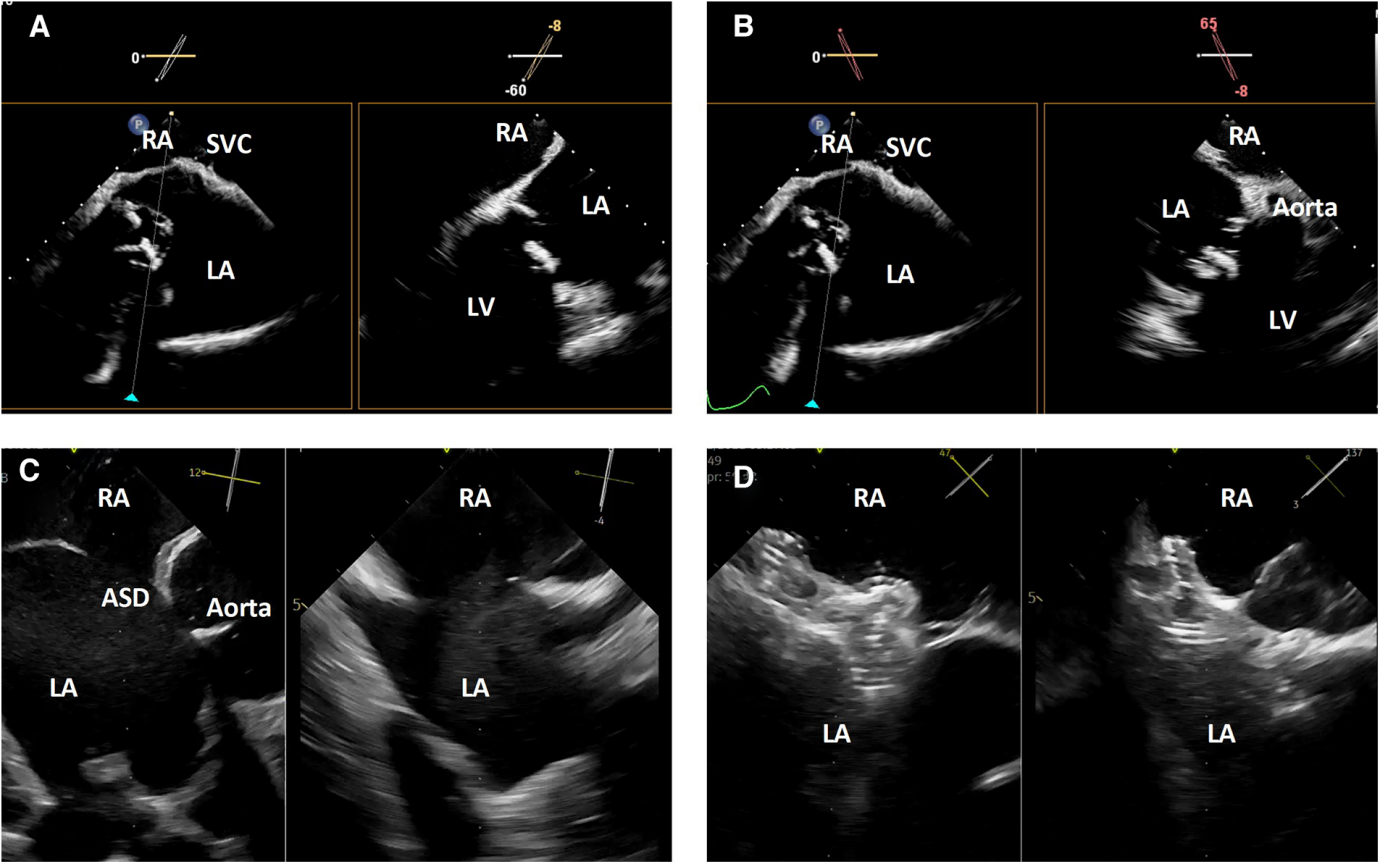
3-dimensional intracardiac echocardiography for transcatheter atrial septal evaluation and interventions. (A) Biplane intracardiac echocardiography evaluation of the interatrial septum in bicaval-like (left) and 4-chamber-like (right) views; (B) biplane intracardiac echocardiography evaluation of the interatrial septum in bicaval-like (left) and aortic short axis (right) views; (C) biplane intracardiac echocardiography evaluation of an atrial septal defect in aortic short axis (left) and orthogonal (right) views; (D) biplane intracardiac echocardiographic guidance of a transcatheter atrial septal defect closure. RA, right atrium, SVC, superior vena cava; LA, left atrium; LV, left ventricle; ASD, atrial septal defect.
